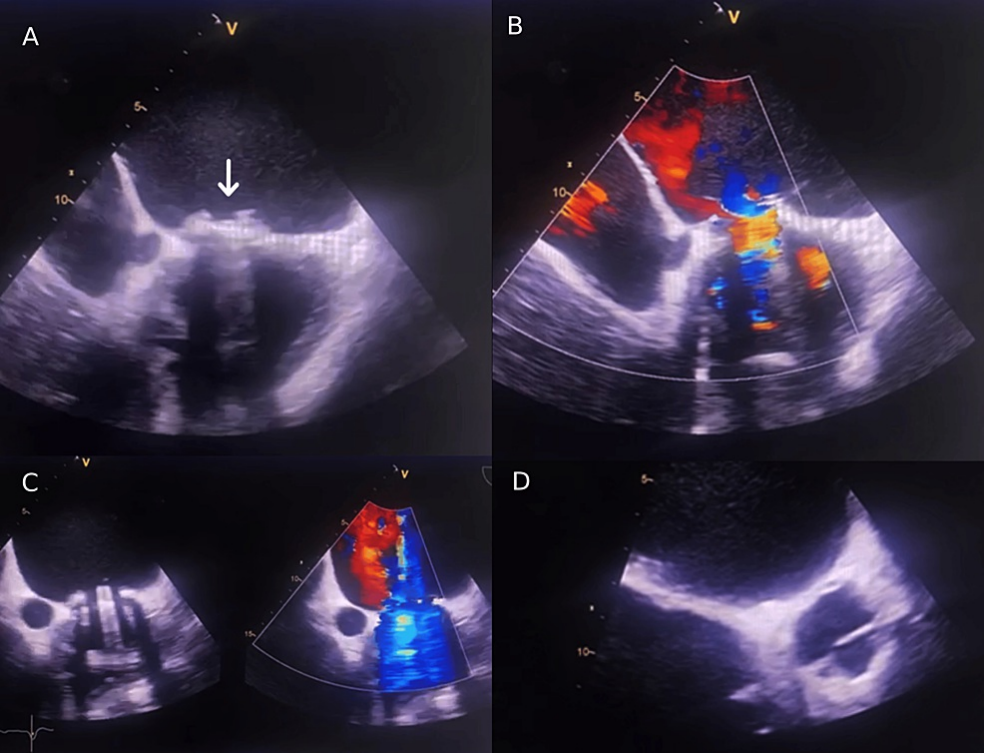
Transesophageal echocardiography showing vegetations on a prosthetic mitral valve (A) with significant periprosthetic leakage (B, C, and D)
